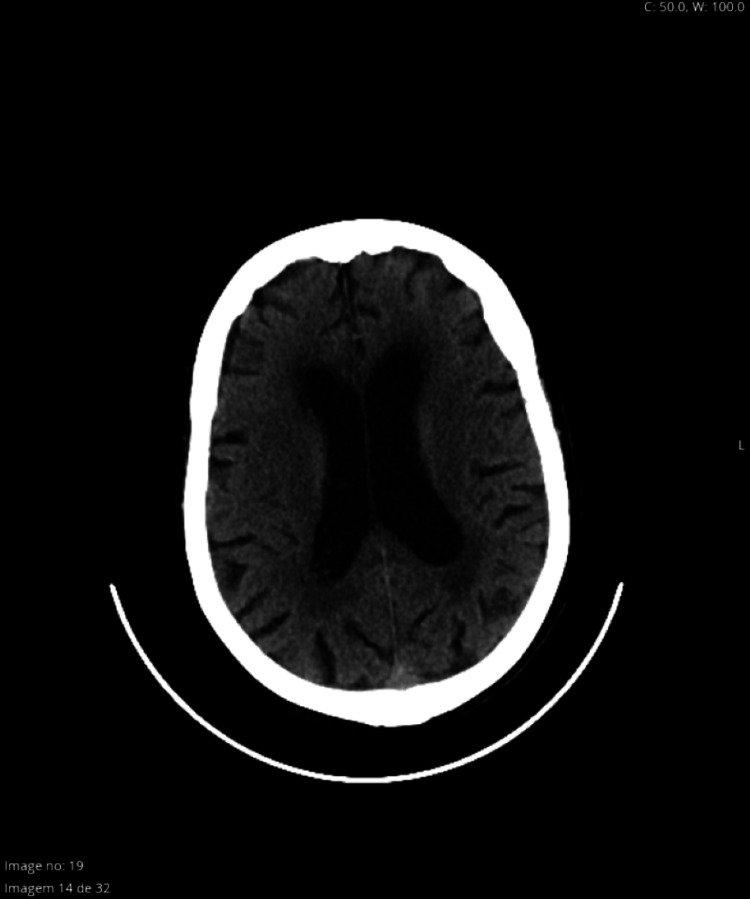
Cranioencephalic computed tomography shows no alterations
